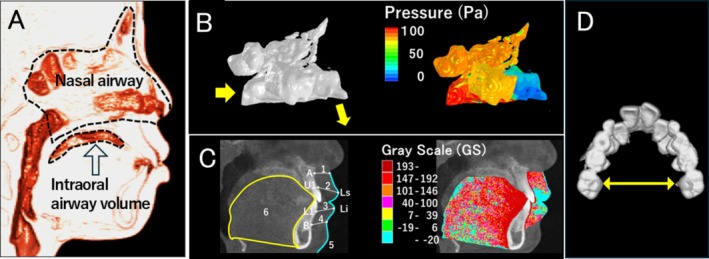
Measurement of nasal airway computed fluid dynamics, muscle‐fat tissue analysis, and maxillary dental arch width performed in this study. (A) Constructing 3D images from CBCT data and extracting models of the nasal airway, intraoral airway, and maxillary dental arch width. (B) Computed fluid dynamics in the nasal airway using the 3D nasal airway model, displaying pressure values to evaluate nasal ventilation condition. (C) Image mapping of muscle‐fat tissue corresponding to the upper and lower lips, the upper and lower orbicularis oris, and tongue in the sagittal midface from CBCT data. Line 1: Straight line from Point A to the base of the nose. Line 2: Straight line from the cervical region of the maxillary central incisor (U1) to the most protruding point of the upper lip (Ls). Line 3: Straight line from the cervical region of the mandibular central incisor (L1) to the most protruding point of the lower lip (Li). Line 4: Straight line from point B to the mental‐labial groove. Line 5; Soft tissue contour (light blue solid line). Line 6; Tongue contour (yellow solid line). Upper orbicularis oris; Between line 1 and line 2. Upper lip; Between line 2 and the lower edge of the upper lip, Lower lip; Between line 3 and the upper edge of the lower lip. Lower upper orbicularis oris; Between line 3 and line 4. (D) Measurement of maxillary dental arch width: Inter first‐molar distance.
